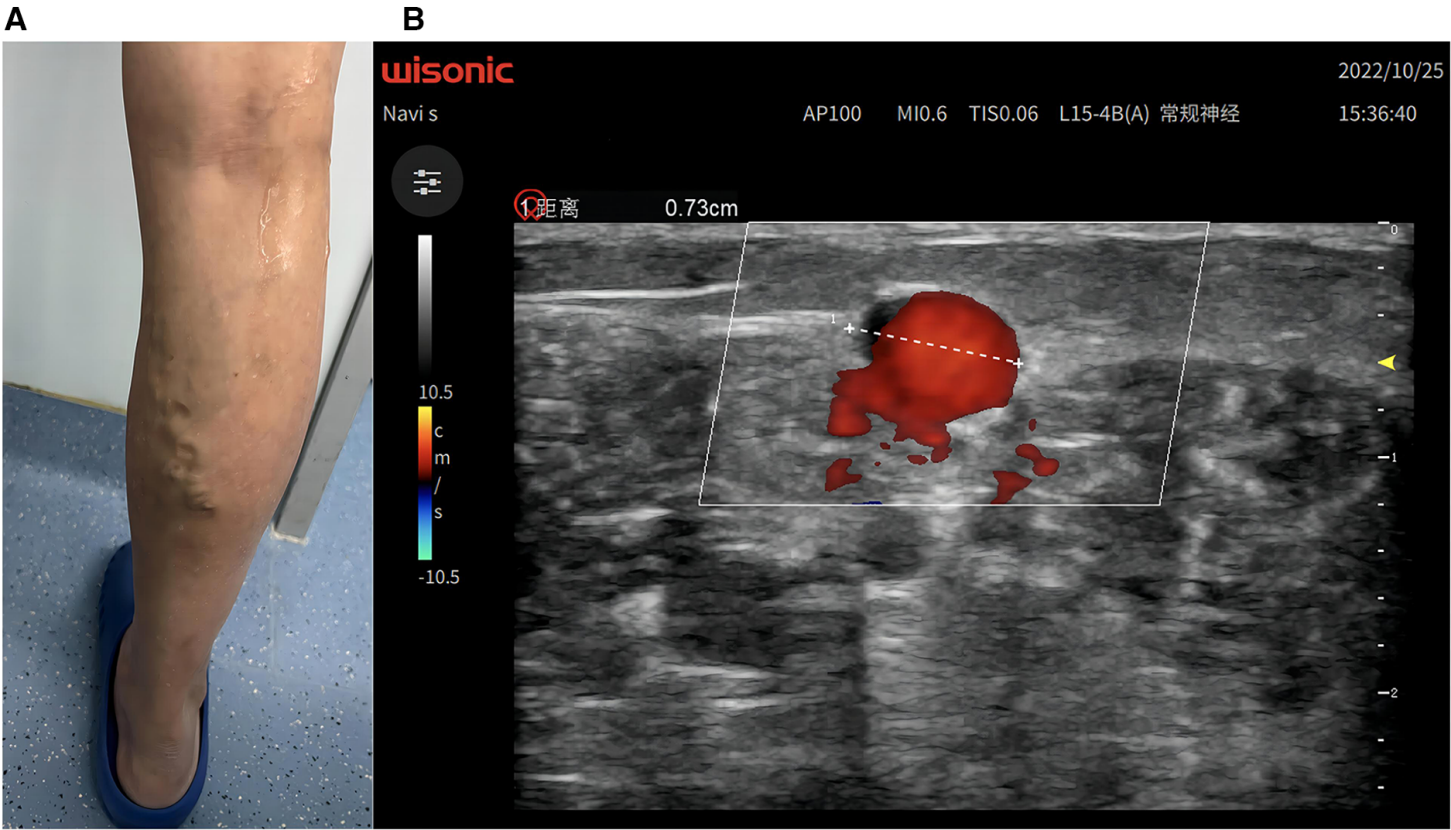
(A) Appearance before operation. (B) Preoperative ultrasound showed small saphenous vein regurgitation.
